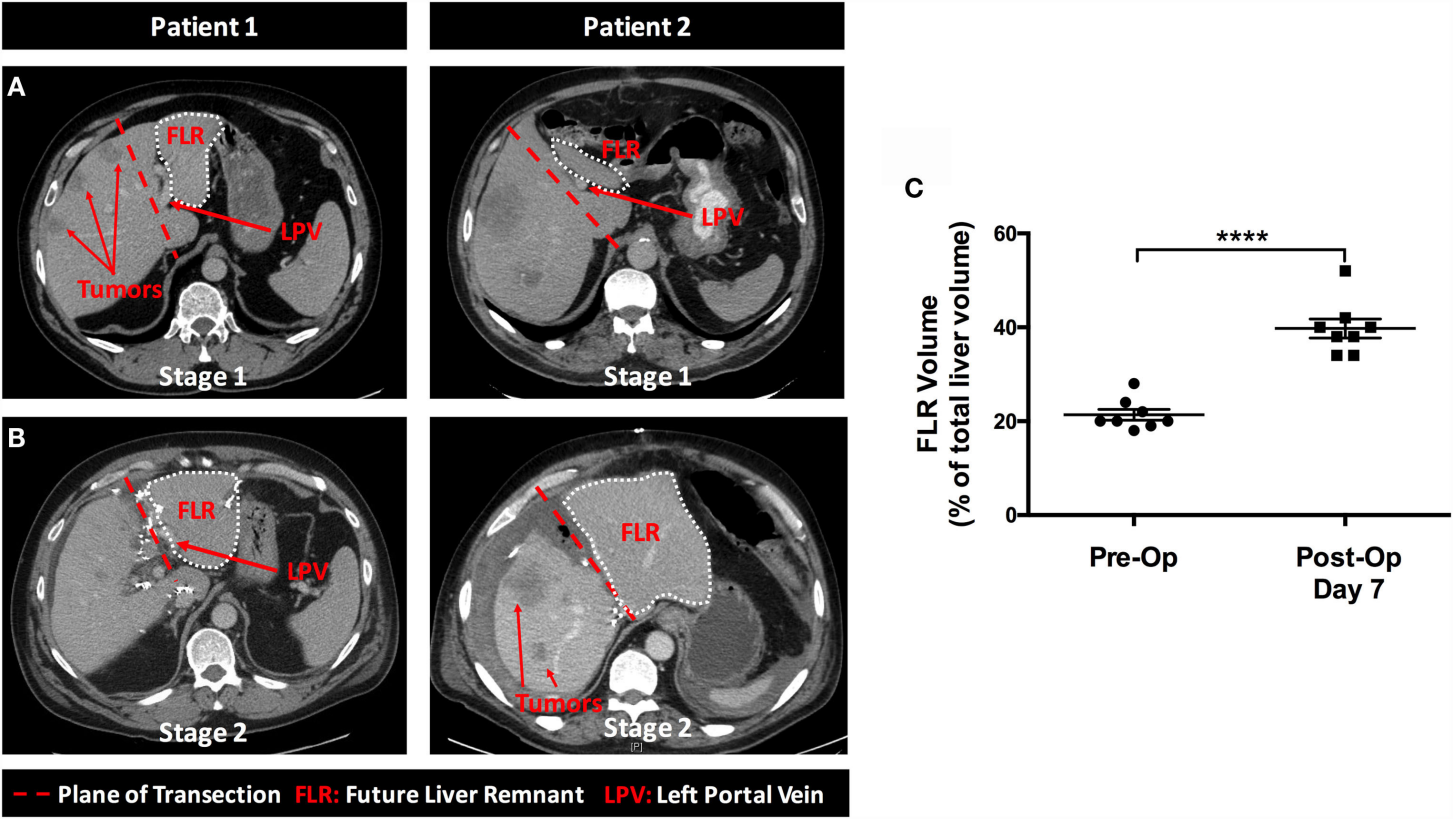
Rapid hypertrophy of the future liver remnant occurs between stages 1 and 2 of the ALPPS procedure. In Figure 2, we present a series of representative cross-sectional CT images taken at various stages throughout the ALPPS procedure. (A) The burden of malignancy within the liver at the time of diagnosis; (B) the magnitude of liver hypertrophy observed following ALPPS Stage 1; and (C) a numerical summary of the change in volume for the FLR (expressed as a percentage of total liver volume) pre- and post-ALPPS, as determined by CT volumetry for eight patients.
